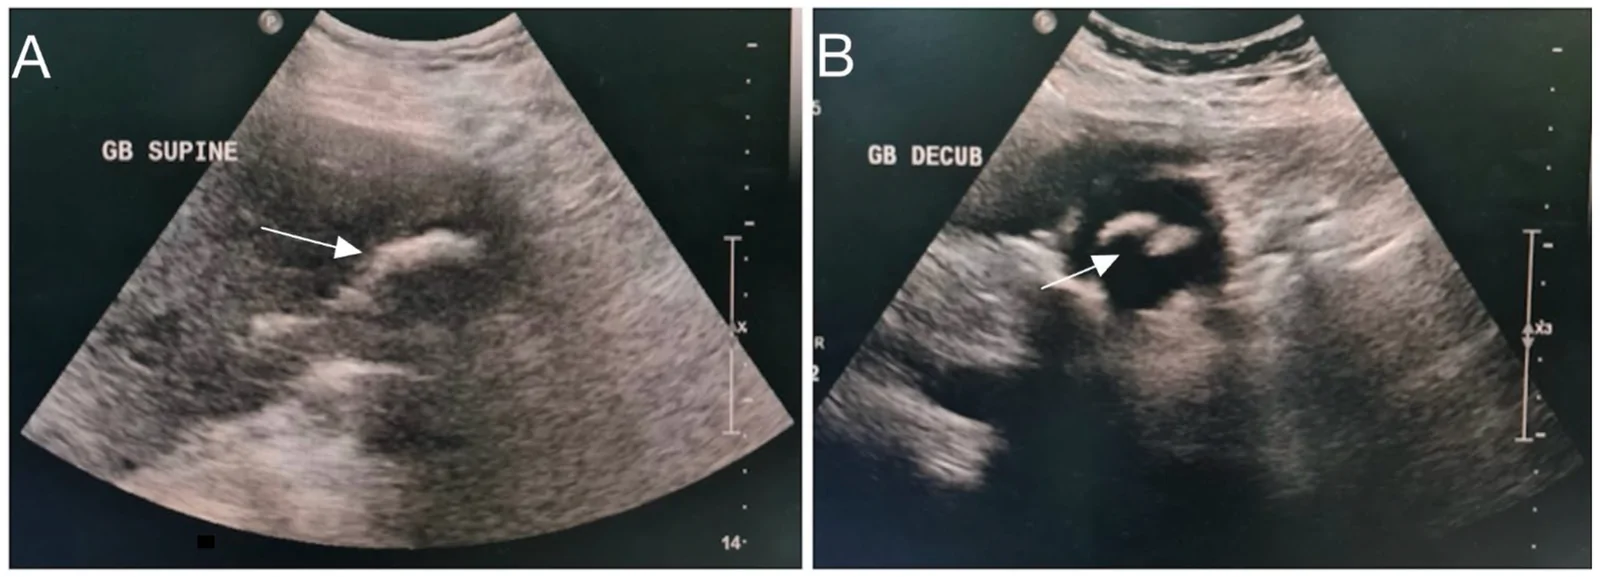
Ultrasonography detected gallstones (arrow). (A) Supine position showing the presence of a gallstone, (B) decubitus position confirming the same stone with a different angle of view.
